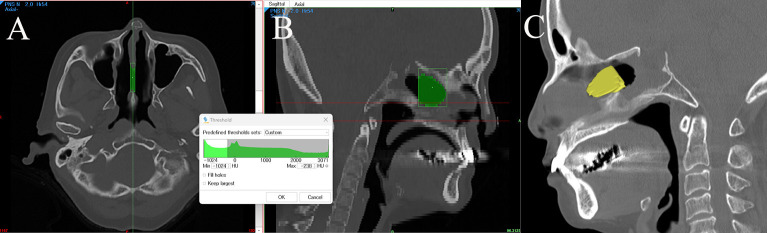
Quantification of the resected posterior septal area using computed tomography. A. Reconstructed image of the resected area (yellow) in a sagittal view of the nasal septum. B. Measuring the extent of posterior septectomy using Mimics software.
